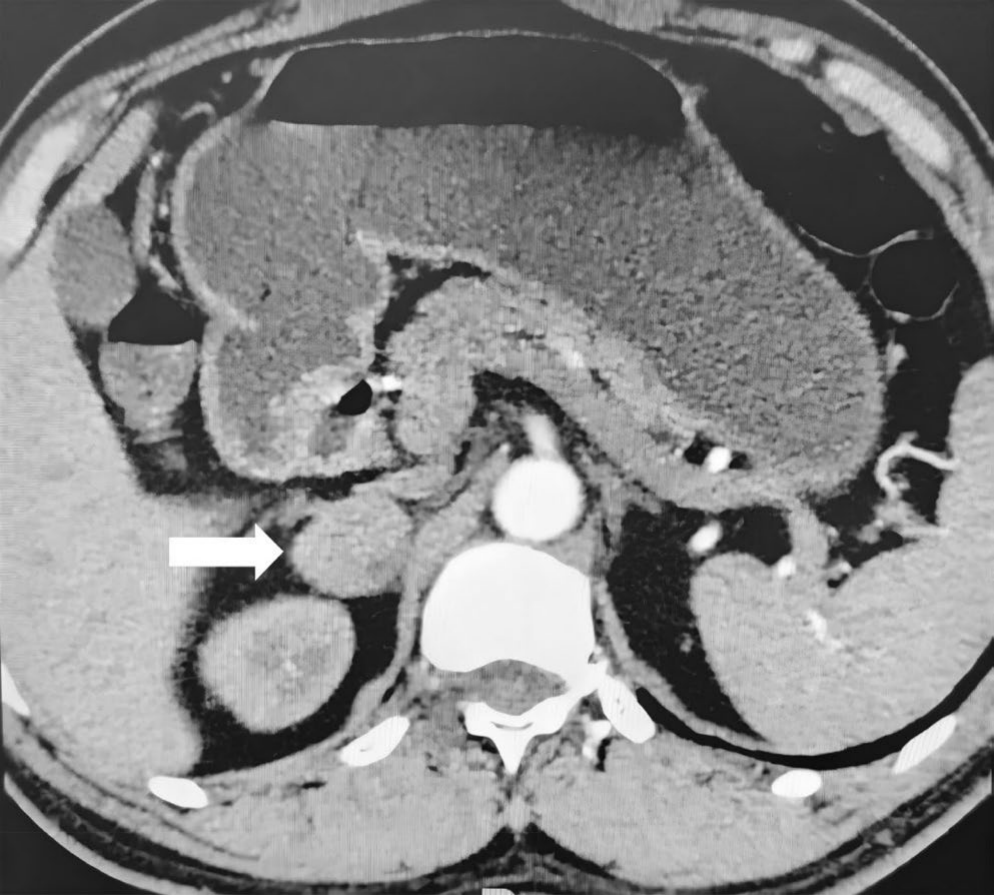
The axial CT image reveals a clearly demarcated mass (indicated by the white arrow) located in the right adrenal gland region.
